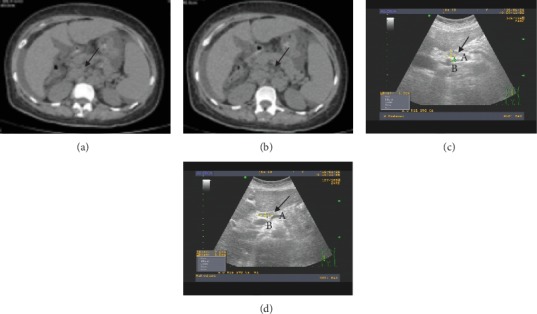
Enlarged lymph node (LN) was detected in autoimmune liver disease (AILD). (a) Abdominal CT examination indicated the enlargement of para-aortic lymph nodes. (b) Abdominal CT examination showed the enlargement of pancreatic lymph nodes. (c) Abdominal ultrasonography showed round enlarged lymph nodes around the pancreas. (d) Abdominal ultrasonography revealed oval enlarged lymph nodes around the pancreas. Abdominal lymph nodes larger than 5 mm but at their shortest diameter were counted. The arrow points to the lymph node: (A) longest axis and (B) perpendicular axis.
